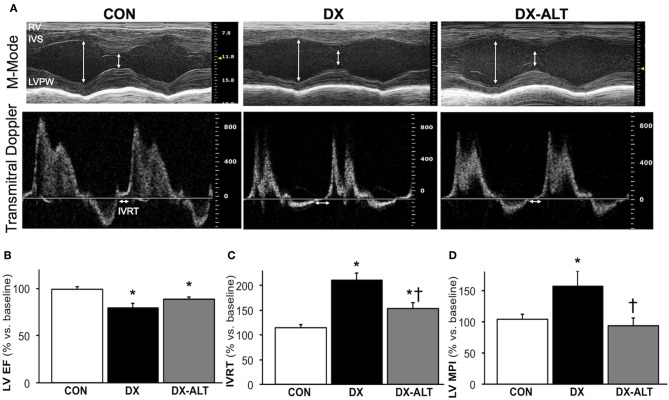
ALT-711 therapy partially alleviated diabetic cardiomyopathy by primarily improving Doppler-derived parameters of diastolic function. (A) A representative paired M-mode echocardiograms (*top panel*) and transmitral Doppler flow (*bottom panel*) at 8 weeks of treatment in control (Con), untreated diabetic (DX), and treated diabetic (DX-ALT) groups. IVS, interventricular septum; LVPW, left ventricular posterior wall; RV, right ventricle; IVRT: isovolumic relaxation time. (B) Mean ± SE of percentage change after 8 weeks of treatment compared to baseline value for ejection fraction (EF) of the left ventricle (LV) in control (Con), untreated diabetic (DX), and treated diabetic (DX-ALT) groups. (C) Mean ± SE of percentage change at 8 weeks compared to baseline value for isovolumic relaxation time (IVRT) of the left ventricle in control (Con), untreated diabetic (DX), and treated diabetic (DX-ALT) groups. (D) Mean ± SE of percentage change at 8 weeks compared to baseline value for myocardial performance index (MPI) in control (Con), untreated diabetic (DX), and treated diabetic (DX-ALT) groups. *n* = 9 − 11/group, ^*^*P* < 0.05 when comparing values from age-matched controls. ^†^*P* < 0.05 when comparing values from DX group.
